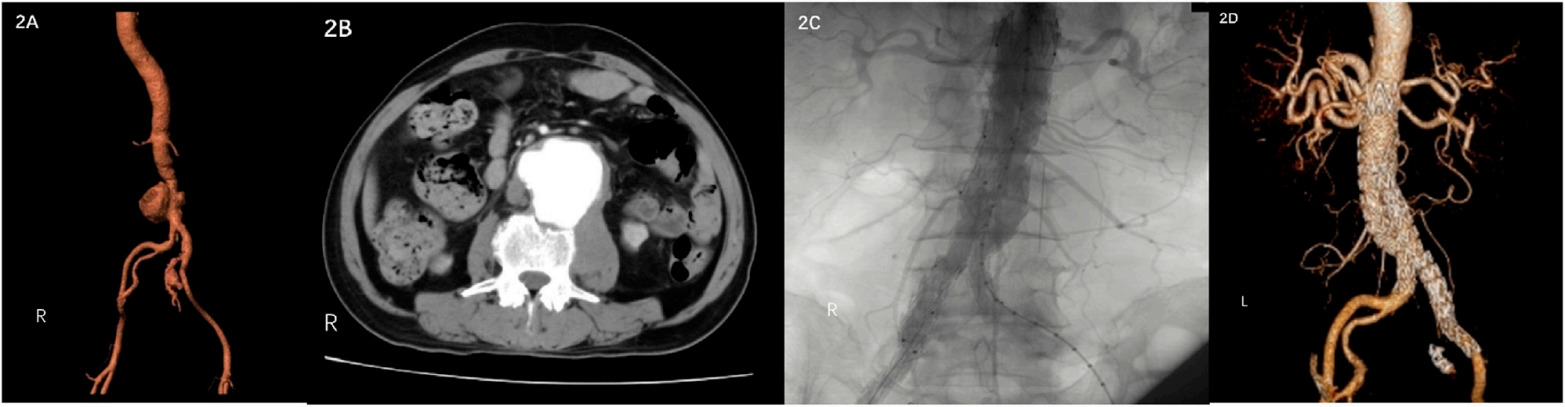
(A) (B) Contrast-enhanced CT showed pseudoaneurysm formation in the middle and lower abdominal aorta and right internal iliac artery. (C) DSA showed no endoleaks. (D) CTA was reexamined 5 months after the operation.
